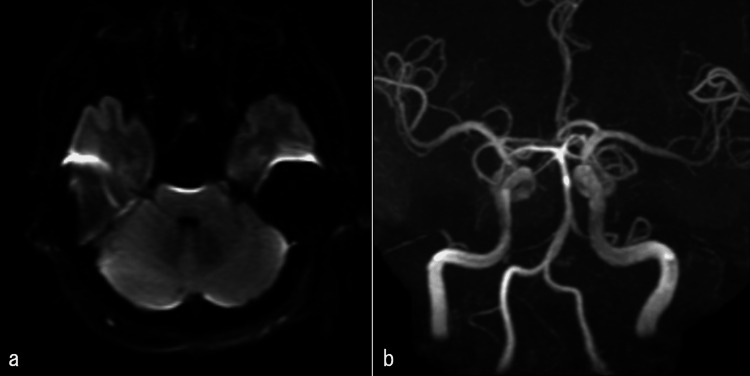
Head magnetic resonance imaging and angiography on the next day of the surgery a) Head magnetic resonance imaging (diffusion-weighted imaging): No obvious acute ischemia. b) Head magnetic resonance angiography: No obvious vascular occlusion.
